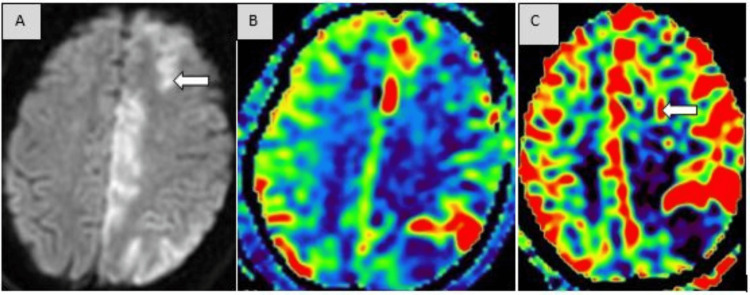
Showing infarct in ACA-MCA watershed infarct with restoration of perfusion on timely thrombolysis. (A) MRI brain axial sections on DWI show an acute ischemic infarct in the left fronto-parietal region (arrow) involving ACA-MCA territory. (B) ASL image showing a large ischemic penumbra in the left fronto-parietal lobe. (C) Effective restoration of perfusion (arrow) after thrombolysis in the previously hypoperfused ischemic penumbra.
